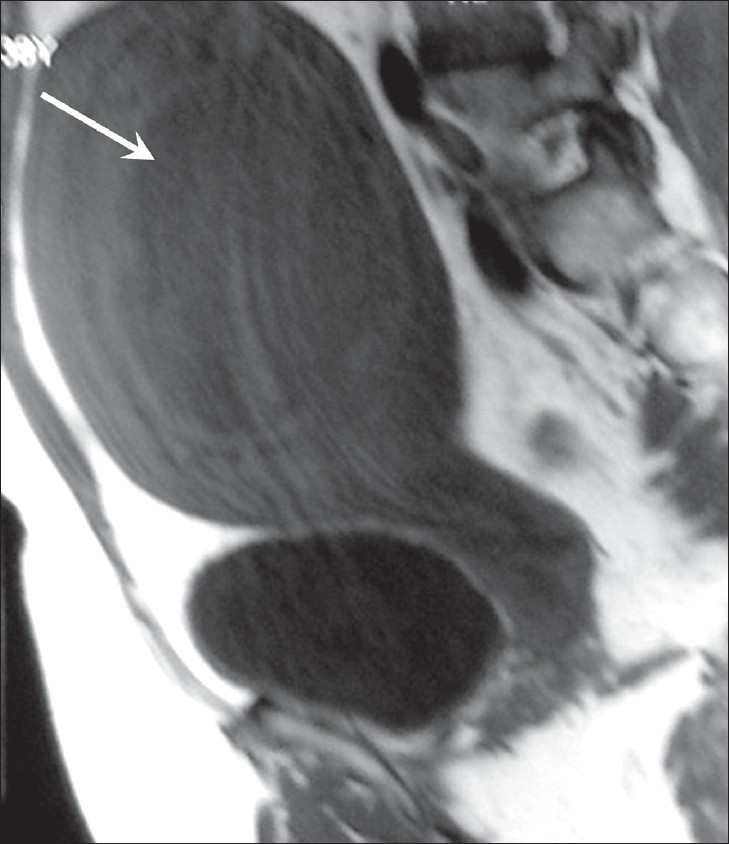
Sagittal T1W MRI reveals cystic degeneration in a hypointense intrauterine fibroid (white arrow)
